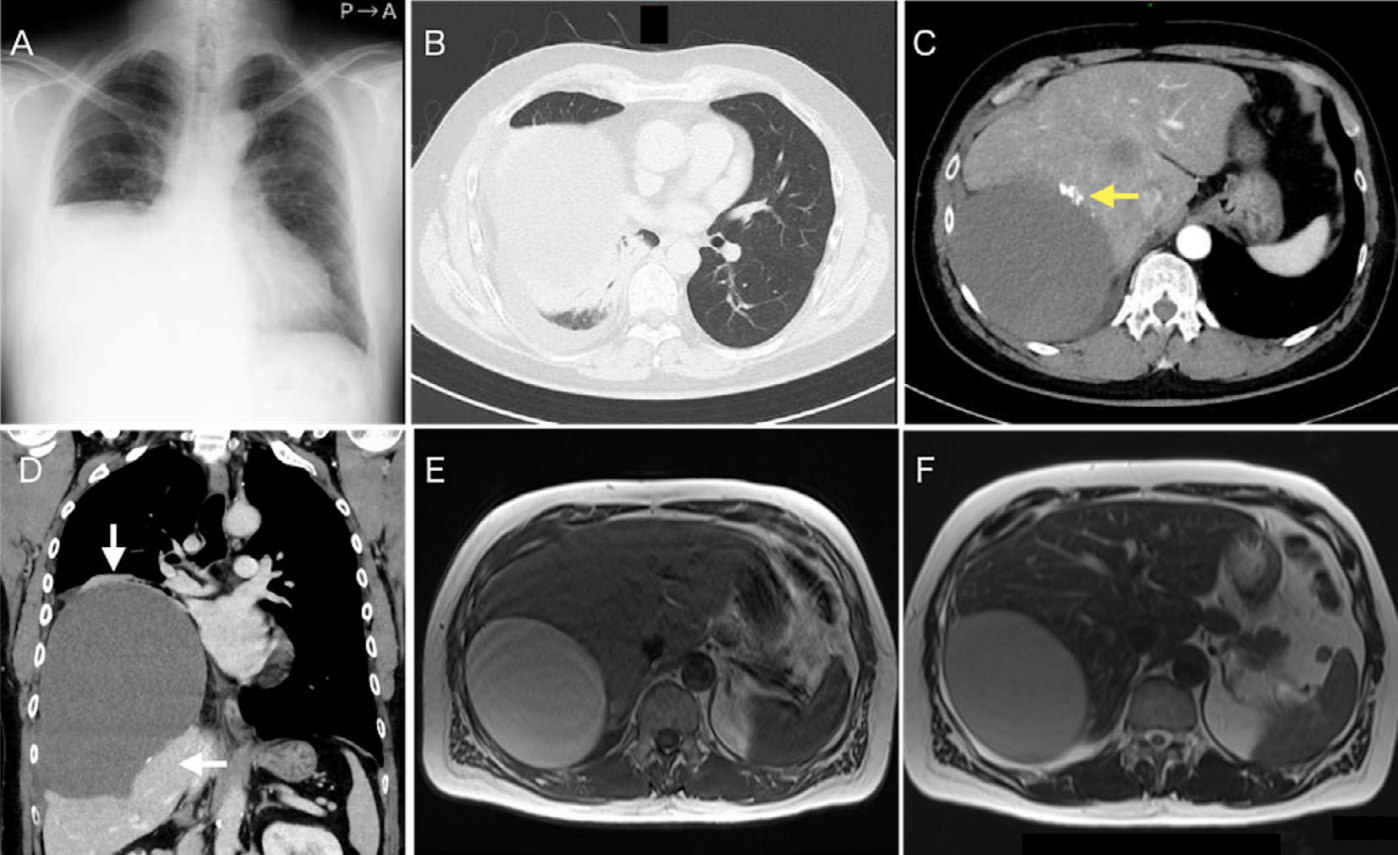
(A) Chest x‐ray shows decreased radiopacity of the right lower lung field. (B–D) Enhanced chest computed tomography images show a cystic mass with no contrast enhancement measuring 18 × 17.5 × 12.8 cm in the right thoracic cavity. Mass effects of the lower lobe of the right lung and liver (white arrow) and partial calcification (yellow arrow) are also revealed. (E, F) Chest magnetic resonance imaging suggests a cystic tumour, but it was difficult to identify until the origin could be determined
